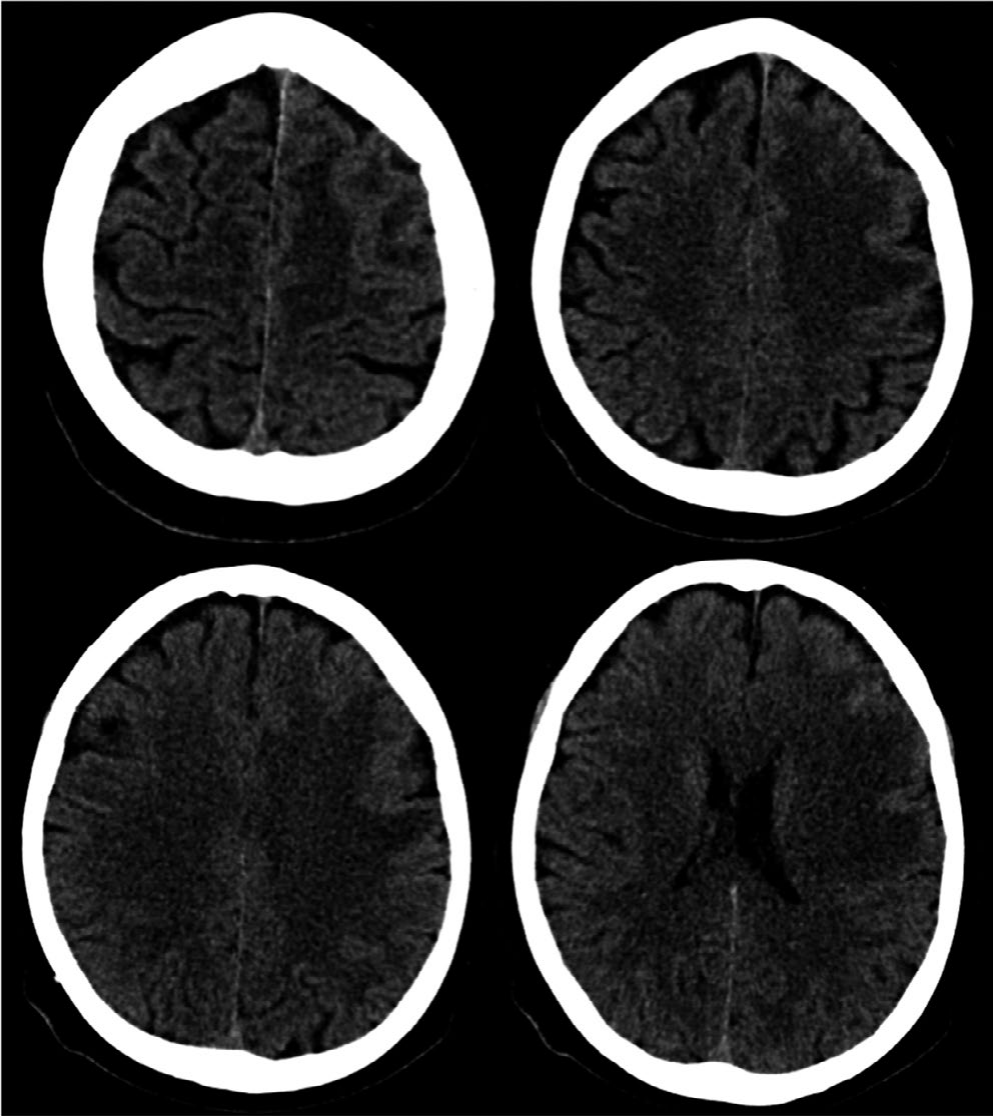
Brain CT performed at the ED, revealing in the left hemisphere an extensive, pale hypodensity with finger‐like borders associated to flattened cerebral sulci*
